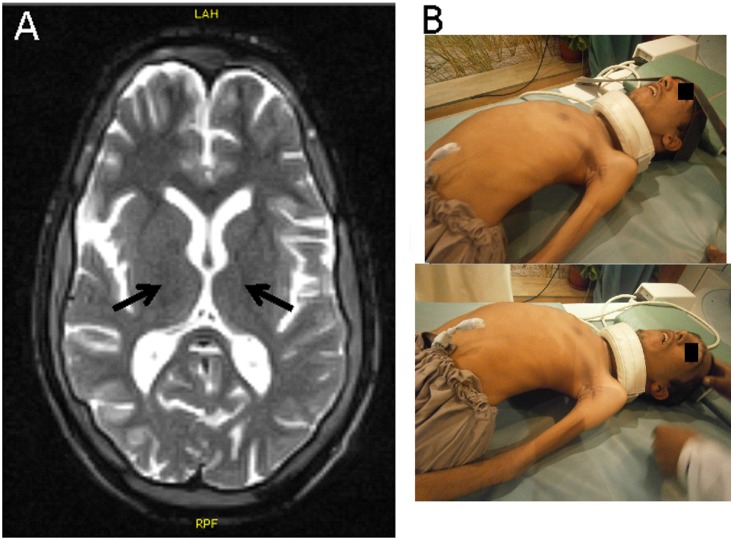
Patient characteristics. a) MRI brain axial T2 weighted sequence showing thinning of the corticospinal tracts (black arrows indicating corticospinal tracts) and b) photographs of one of the patients (IV-4) showing severe scoliosis.
